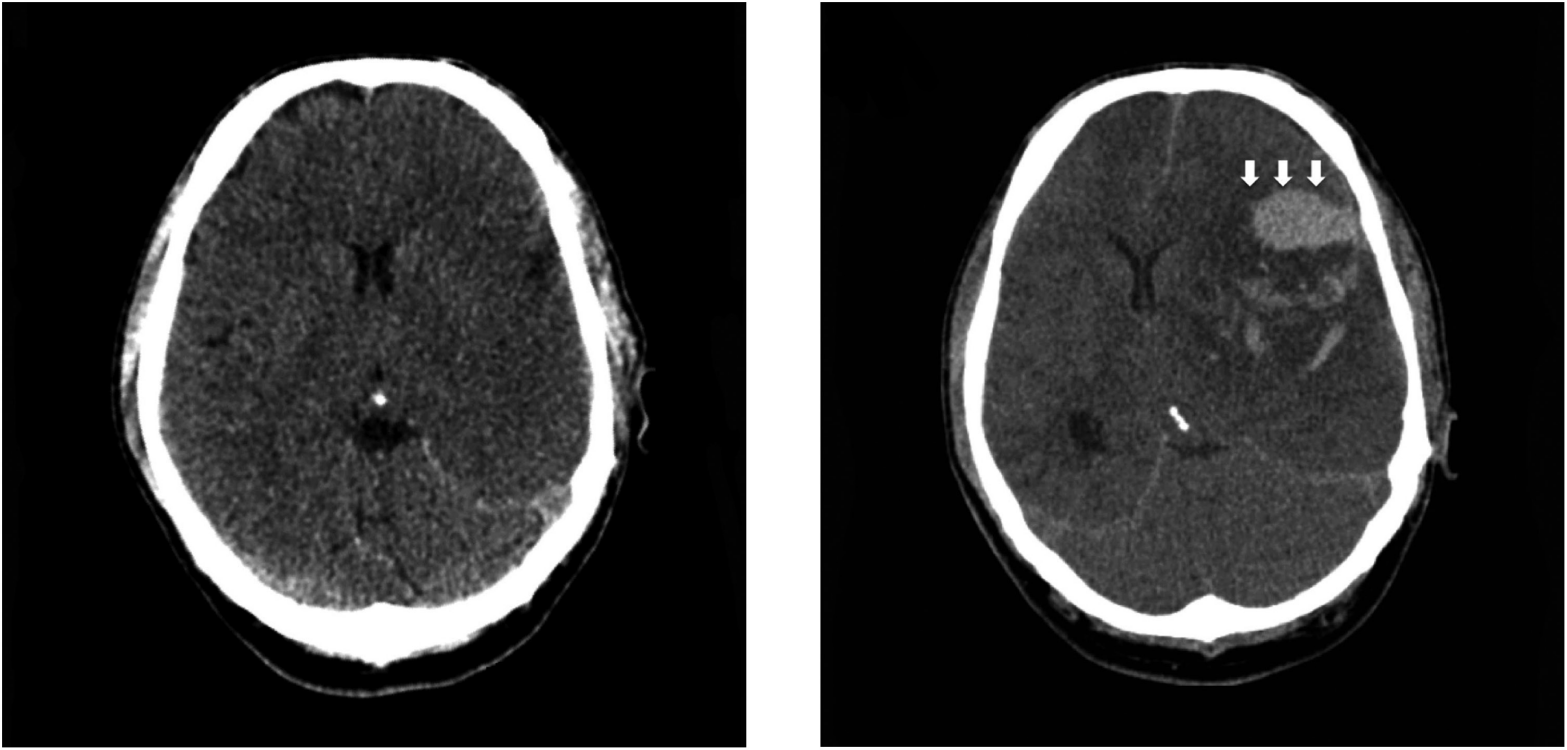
Initial CT head shows extensive loss of grey-white matter differentiation and sulcal effacement affecting the left cerebral hemisphere, consistent with large MCA infarction. Further CT head following neurological deterioration demonstrates haemorrhagic transformation within the infarct (white arrows) with mass effect.
